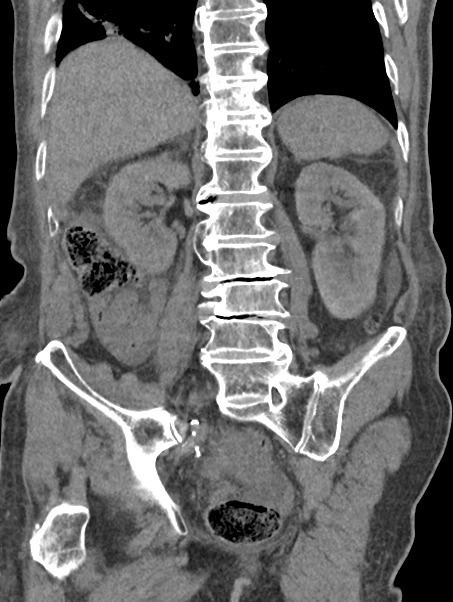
Coronal unenhanced CT image showing that post catheterisation there has been decompression of the grossly distended urinary bladder and a normal left kidney. The urinary bladder is hypertrophied and patulous secondary to chronic obstruction.
